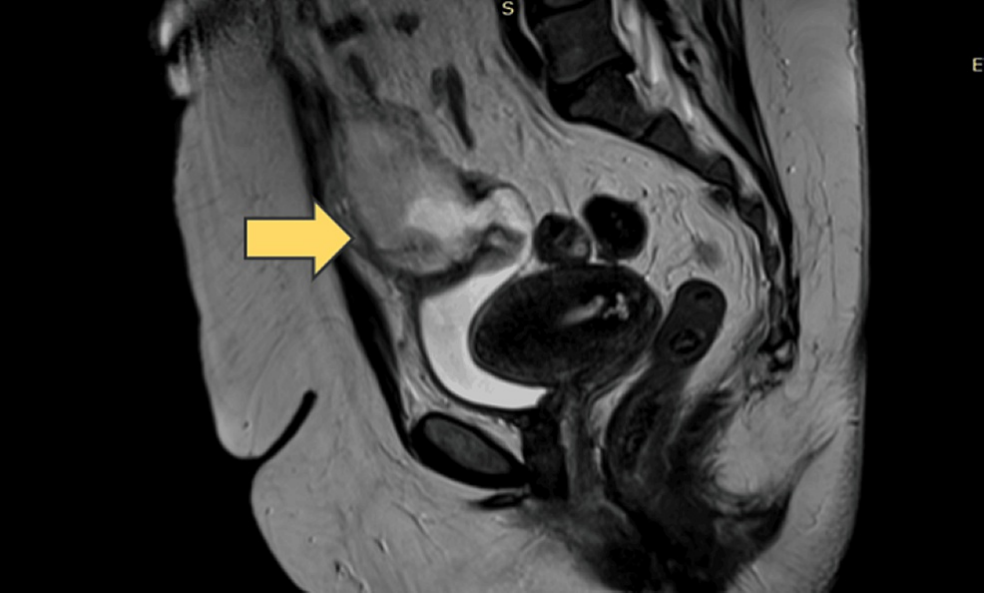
The MRI demonstrated a cystic lesion
